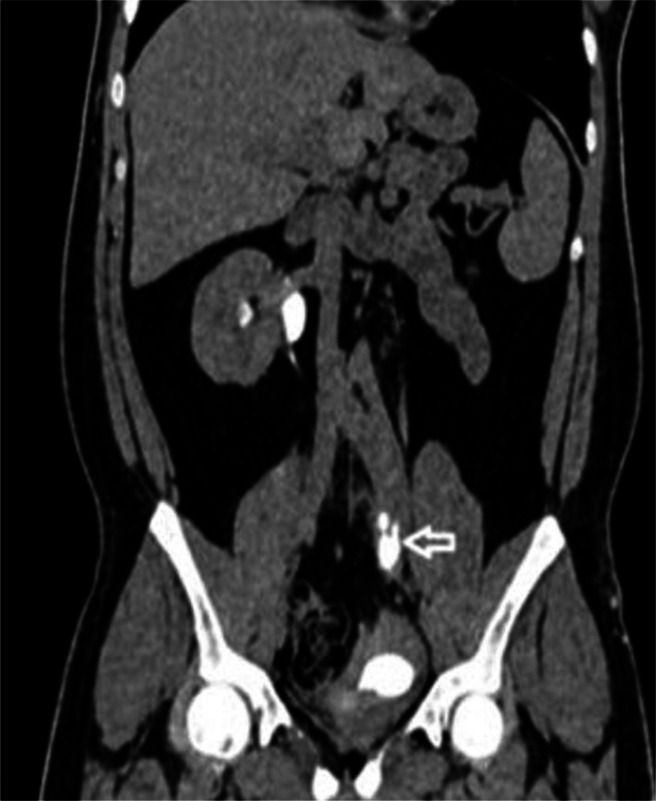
A coronal image of CT urogram depicting hypoplastic left kidney with remnants of left renal tissue showing normal contrast enhancement with delayed excretion into malformed primitive calyceal remnants (annotated by white arrow).
